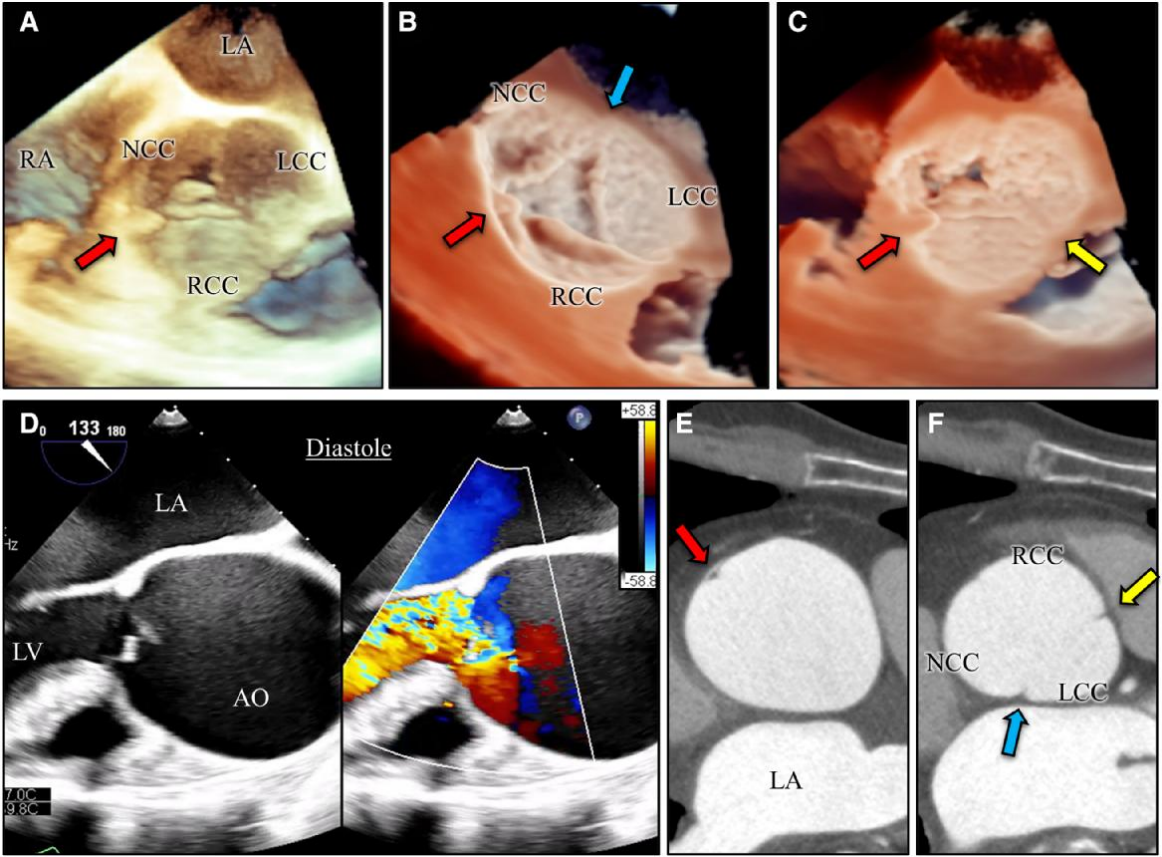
Imaging assessment of aortic valve commissure dissection. Intraoperative transoesophageal echocardiography revealed detachment of the aortic valve commissure between the right coronary cusp and non-coronary cusp (*A*–*C*, lower left arrows). No dissection was found in the other two aortic valve commissures (upper arrow and lower right arrow) (*B* and *C*). Severe aortic regurgitation associated with aortic root enlargement and prolapse of the non-coronary and right coronary cusps of the aortic valve were identified (*D*). Contrast-enhanced computed tomography revealed dissection of the aortic valve commissure from the aortic wall (upper left arrow), but there were no findings suggestive of dissection in the other commissures (lower arrow and upper right arrow) (*E* and *F*). AO, aorta; LA, left atrium; LCC, left coronary cusp; LV, left ventricle; NCC, non-coronary cusp; RA, right atrium; RCC, right coronary cusp.
